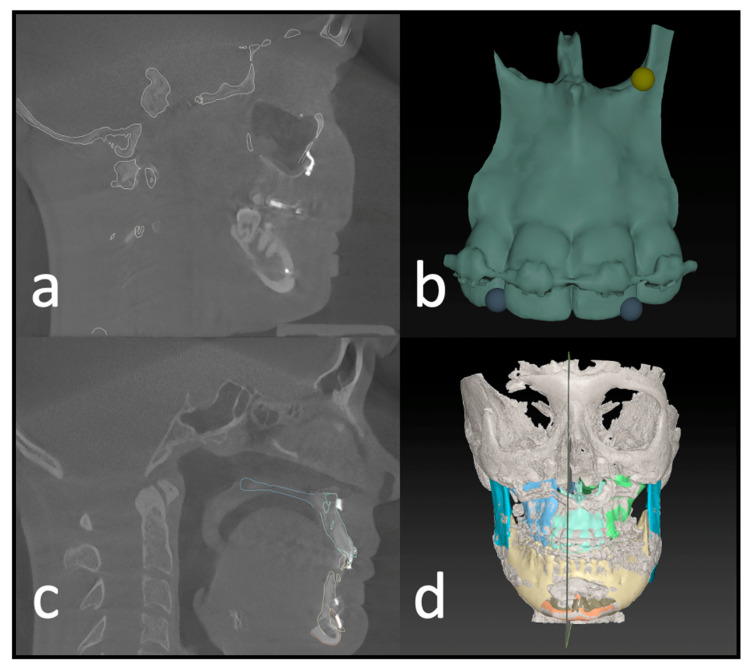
Postoperative analysis of a three-piece maxilla. (a) Matching of the preoperative CT and virtual plan to the postoperative CBCT scan. (b) Identifying landmarks on the segment that can be isolated on the postop CBCT. (c) Visual confirmation of the automatic matching process. (d) Overview of all segments matched to the postop imaging.
